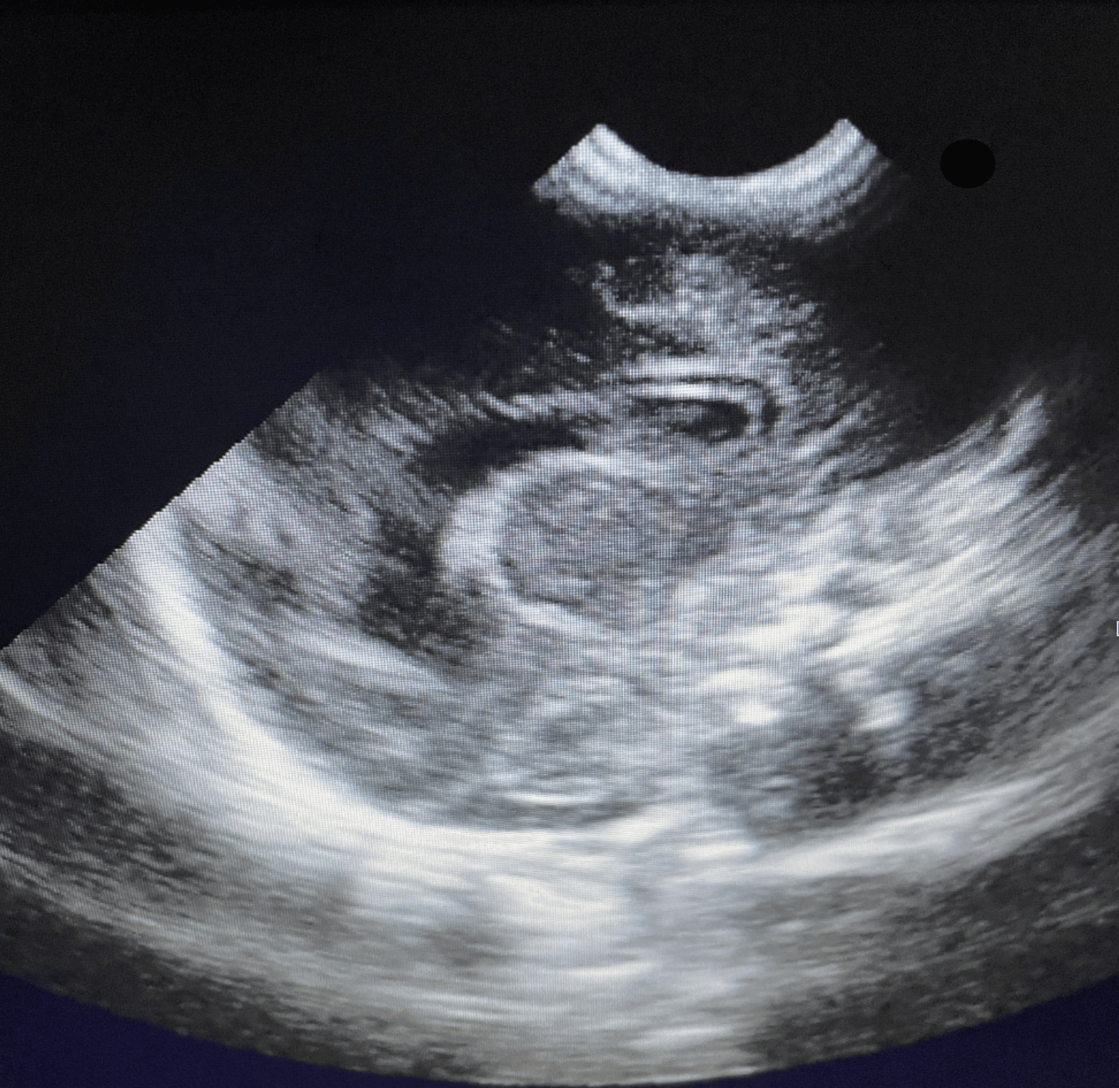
Normal echocardiography of our patient
